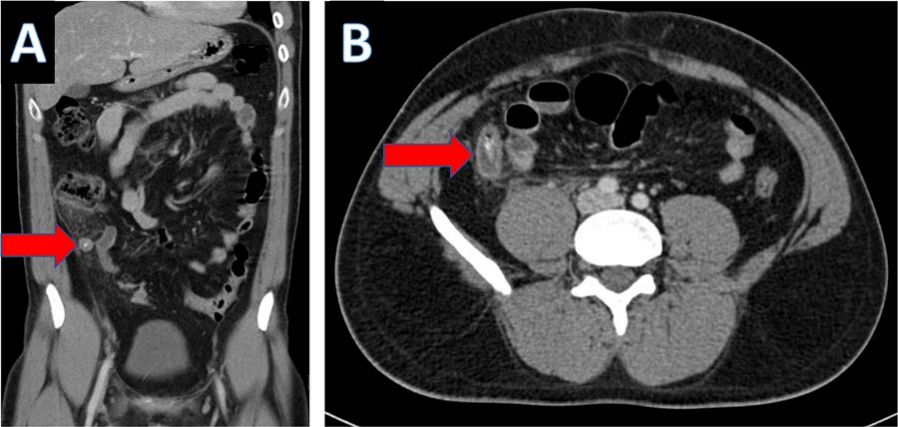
Coronal CT scan showing appendicolith (A) and axial CT showing appendicitis (B).
